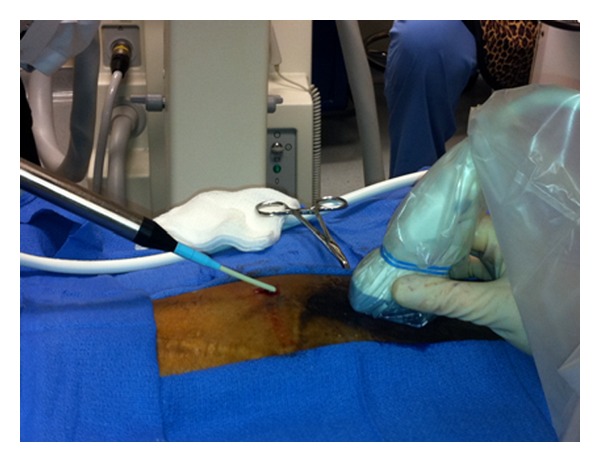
The cryoprobe was inserted via a 12-gauge angiocatheter introducer proximal to the skin graft site of the left lower extremity. The ultrasound probe is being utilized to provide an in-plane view of the cryoprobe and ice ball location during cryoneurolysis treatment.
